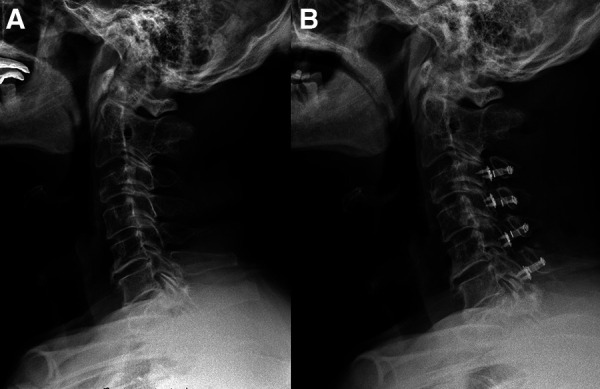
A 63-year-old male patient treated with C4–C7 laminoplasty. (A) Preoperative lateral cervical radiograph (CSVA = 29.5 mm, T1S = 21.8°). (B) Lateral cervical radiograph at final follow-up (18 months after surgery, CSVA = 30.9 mm).
